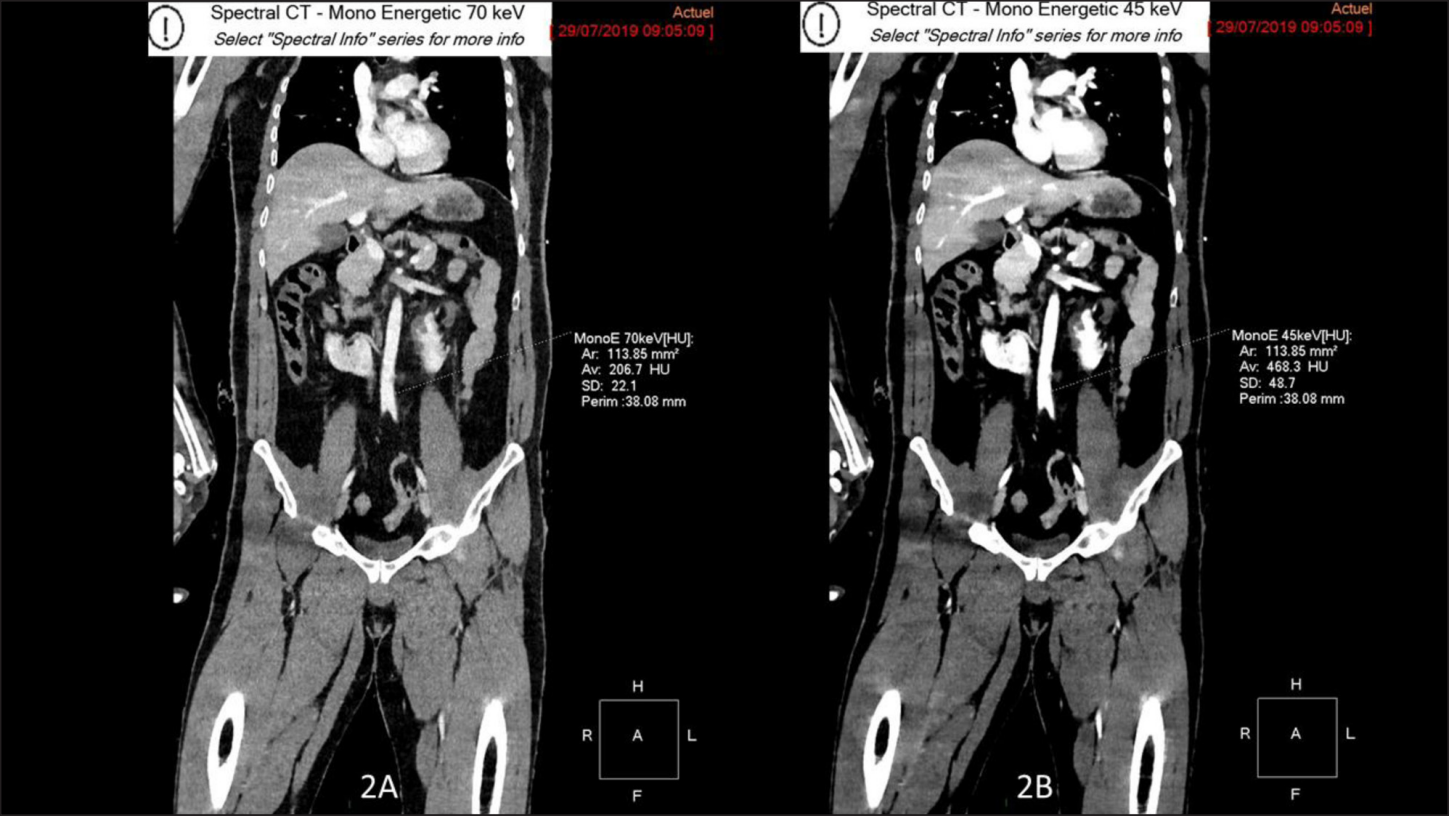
A good enhancement was obtained within the aorta. Intravascular enhancement was better shown at low keV. Three-dimensional reconstruction were performed at 70 keV (equivalent to 120 kVp) (Figure 3A) and at 45 keV (Figure 3B) to confirm the better visualisation of the small visceral arterial branches at low keV. There were no visceral lesions on the two series, but head CT revealed an acute subdural hematoma. The patient was immediately transported to the operating room and deceased within the two hours post-surgery, due to the extensive burned skin lesions.
